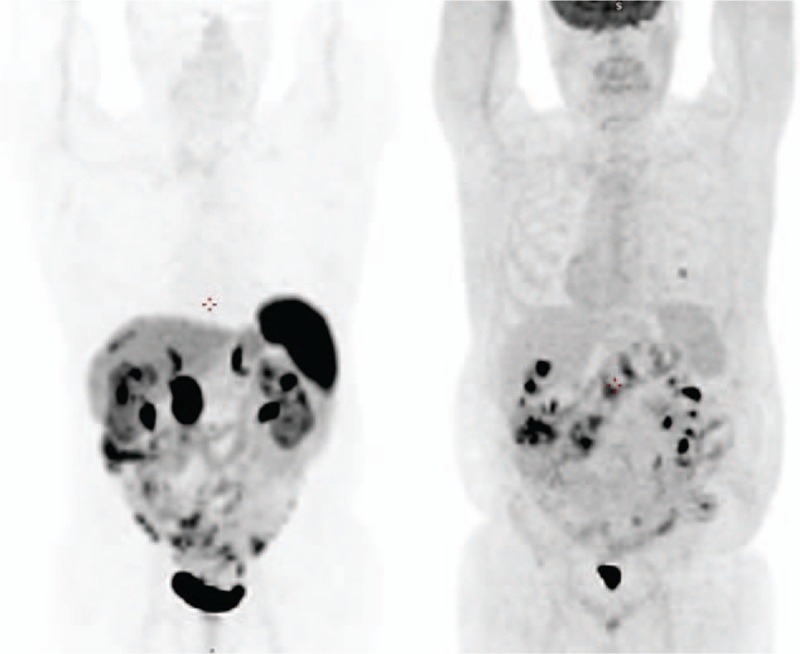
Maximum Intensity Projection (MIP) of ^68^Ga-DOTATOC PET/CT (left) and ^18^F-FDG PET/CT (right) showing the body physiological and pathological distribution of the 2 radiopharmaceuticals: the 2 images can be considered as complementary showing low and intermediate grade lesions (left image) and high grade lesions (right image) that can derive from tumor cell dedifferentiation or, as in the case of the pulmonary nodule, primary.
